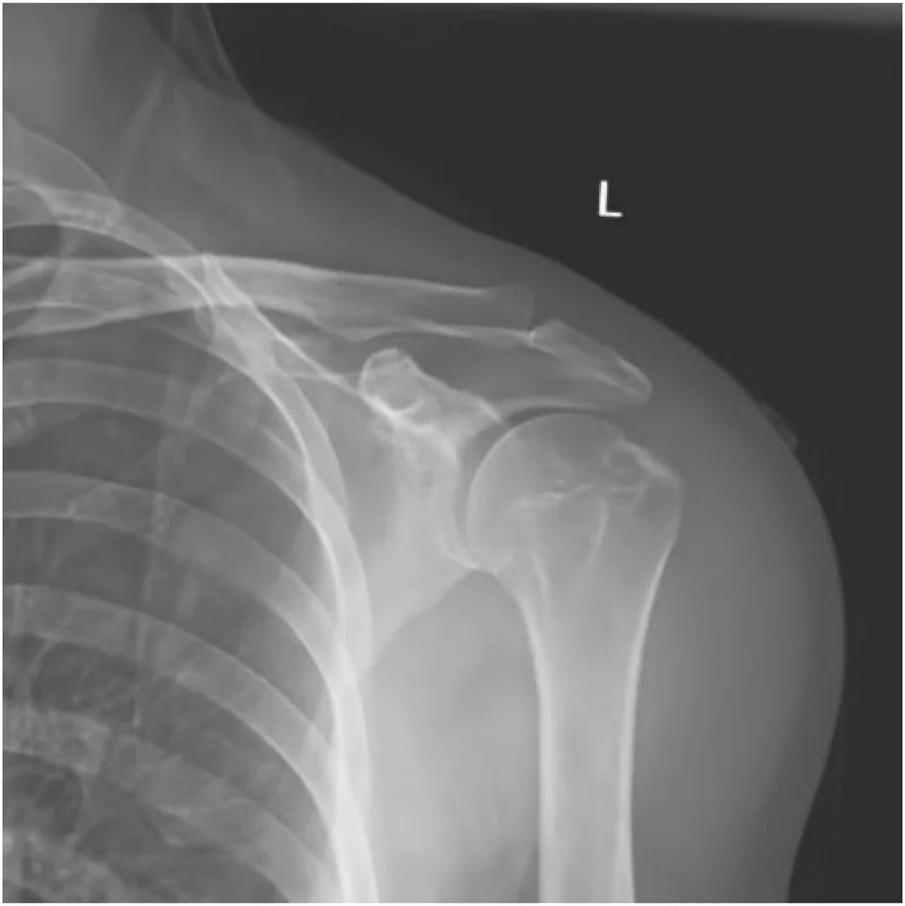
Preoperative left shoulder X-ray of the 58-year-old male patient presented in this case report.
